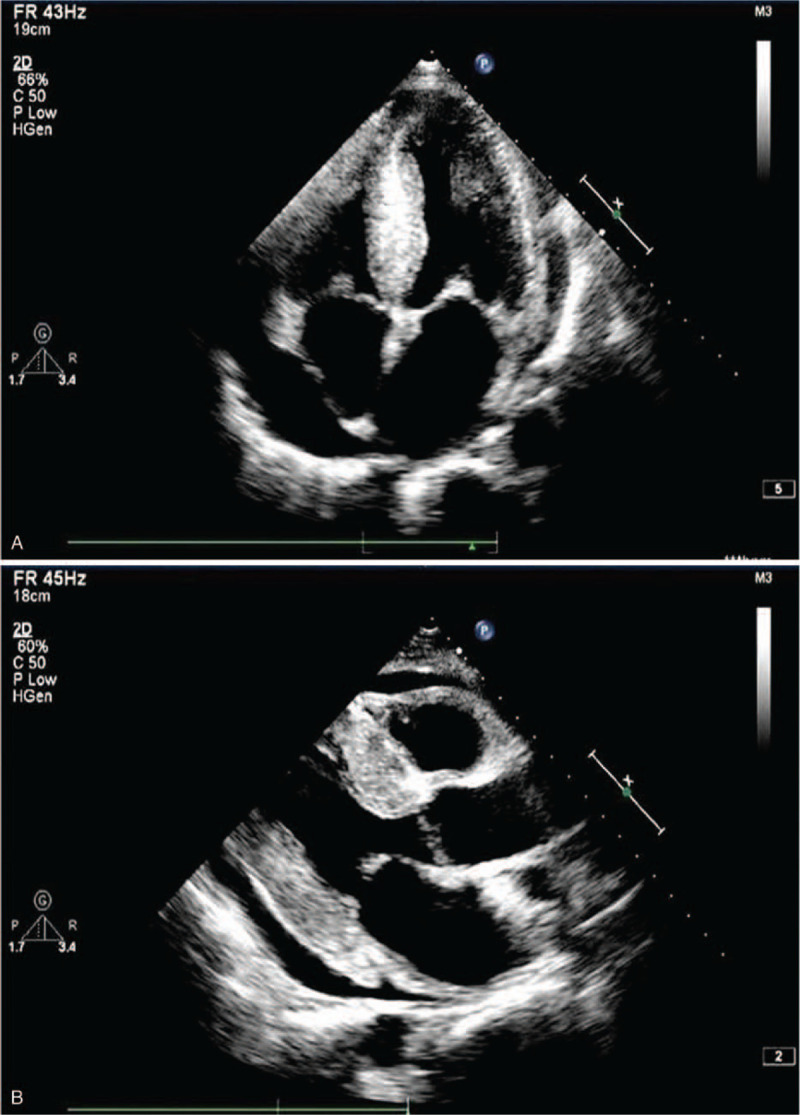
A. Apical Echocardiology. Apical 4 chamber view on echocardiology showed left ventricle hypertrophy, left atrium enlargement, and pericardial effusion. B. Parasternal long axis view echocardiology. Parasternal long axis view echocardiology showed left ventricle concentric hypertrophy and pericardial effusion.
